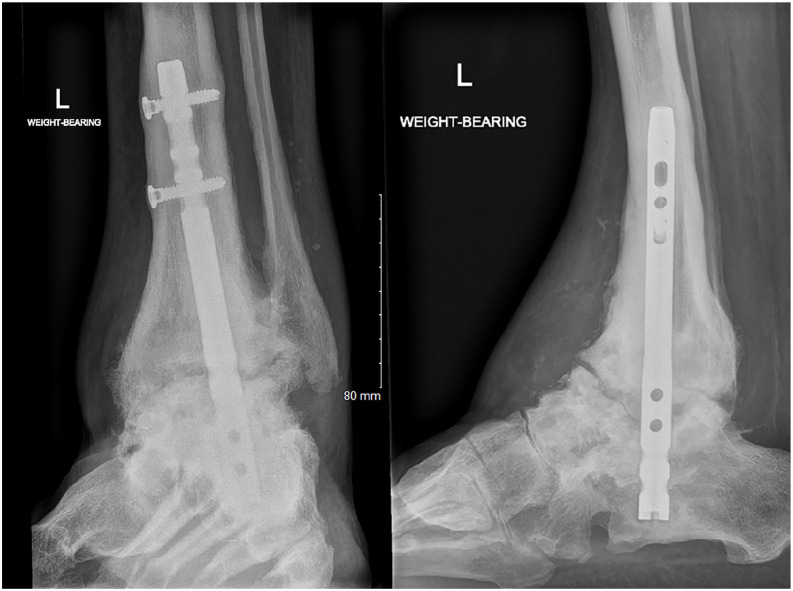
Postoperative radiographs of tibiotalocalcaneal nonunion with restoration of bone stock. (Follow-up imaging of Figure 2.)
